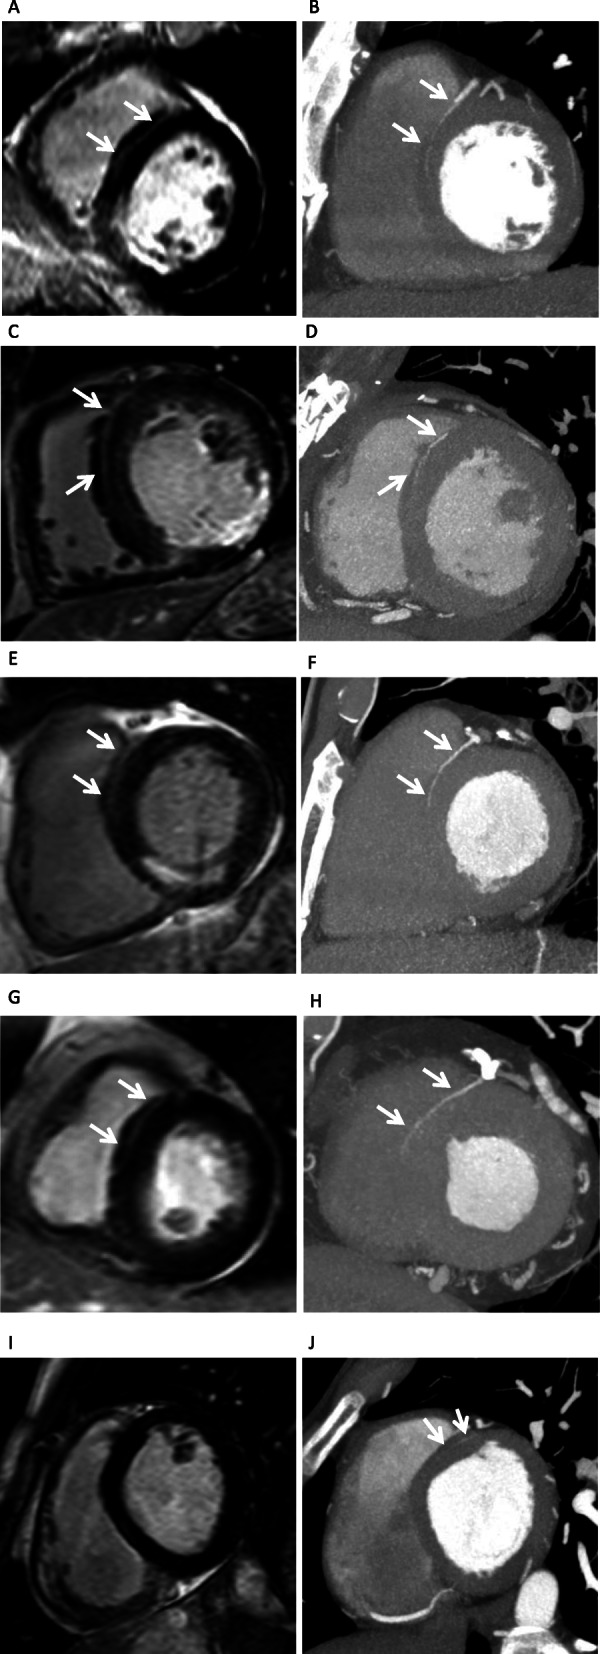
a-j. Some patients of linear high intensity (LHI) in late gadolinium enhancement (LGE) cardiovascular magnetic resonance (CMR) and anterior septal perforator arteries in coronary computed tomography angiography (CorCTA). a-b. Fifty-six-year-old, male, acute inferior myocardial infarction. a LHI on CMR LGE, b anterior septal perforator arteries on CorCTA. C-D. Forty-eight years old, male, old inferior myocardial infarction. c LHI on CMR LGE, d anterior septal perforator arteries on CorCTA. E-F. Fifty-eight-year-old, male, old inferior myocardial infarction. e LHI on CMR LGE, f anterior septal perforator arteries on CorCTA. G-H. Seventy-one-year-old, female, acute anterior myocardial infarction. g LHI on CMR LGE, h anterior septal perforator arteries on CorCTA. i-j. LHI could not be identified (negative LHI) on LGE i. However, CorCTA could detect very thin anterior septal perforator arteries with higher spatial resolution j
